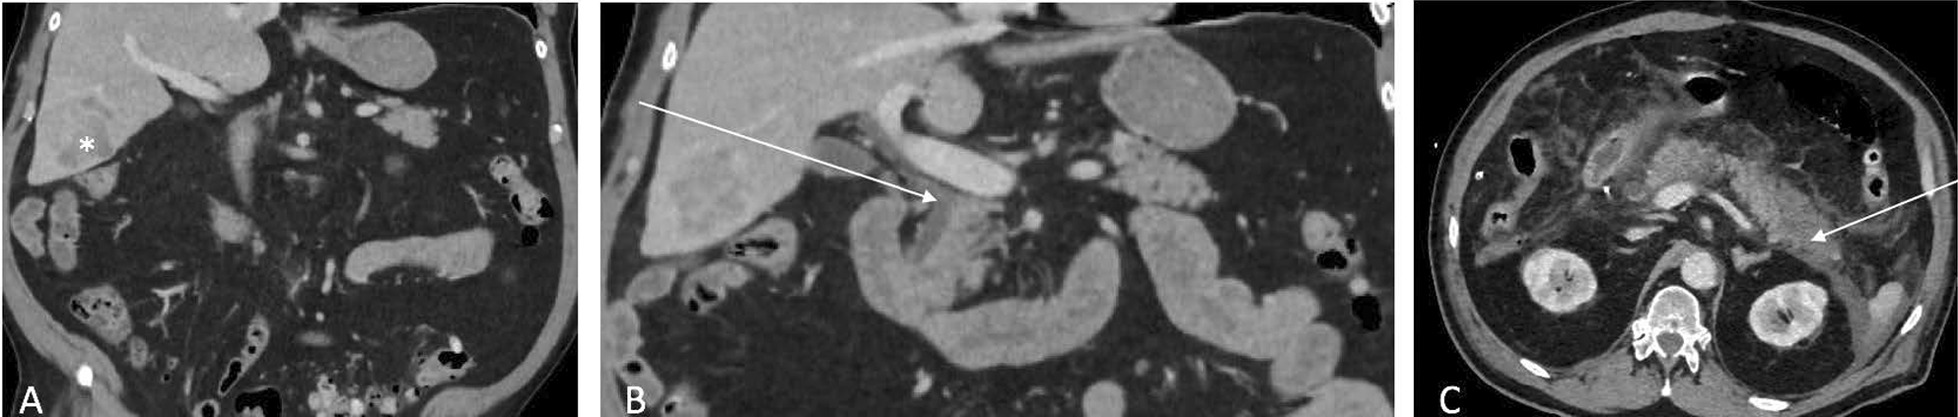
Patient A. A Preoperative CT showing HCC in segment VI (white asterisk). B Preoperative CT, distal implantation of the cystic duct (white arrow). C CT scan at POD 1 showing necrosis of the tail of the pancreas t
